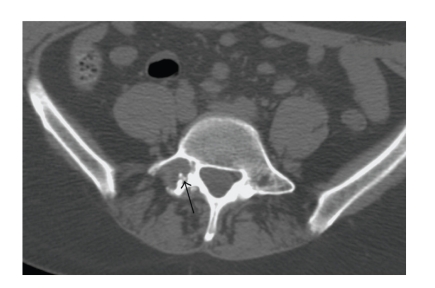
CT image shows a well-circumscribed, minimally expansile lytic lesion in the right pedicle and adjacent transverse process of L5. Tiny densities (arrow) represented bony fragments from adjacent degenerated facet joint.
